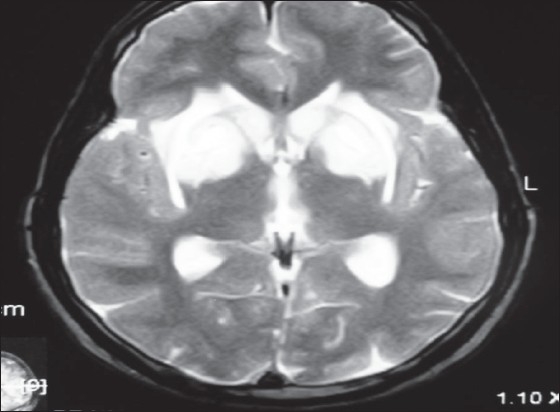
A 24 year old male presented with acute onset headache with drowsiness in the background of recent fever. On examination he had pupil sparing ptosis, vertical gaze paresis, mild sensory neural deafness and bilateral subtle pyramidal signs. MRI brain showed symmetric hyperintense lesions in the caudate nuclei and putamina extending into the thalami and periaqueductal regions, on T2W images (Figure 6). His serum and CSF lactic acid levels were raised.A quadriceps mucle biopsy revealed subsarcoplasmic accumuilation of mitochondria) associated with cytochrome oxidase negative fibres. No mitochondrial mutations were detected. Following intravenous Thiamine therapy he recovered rapidly
